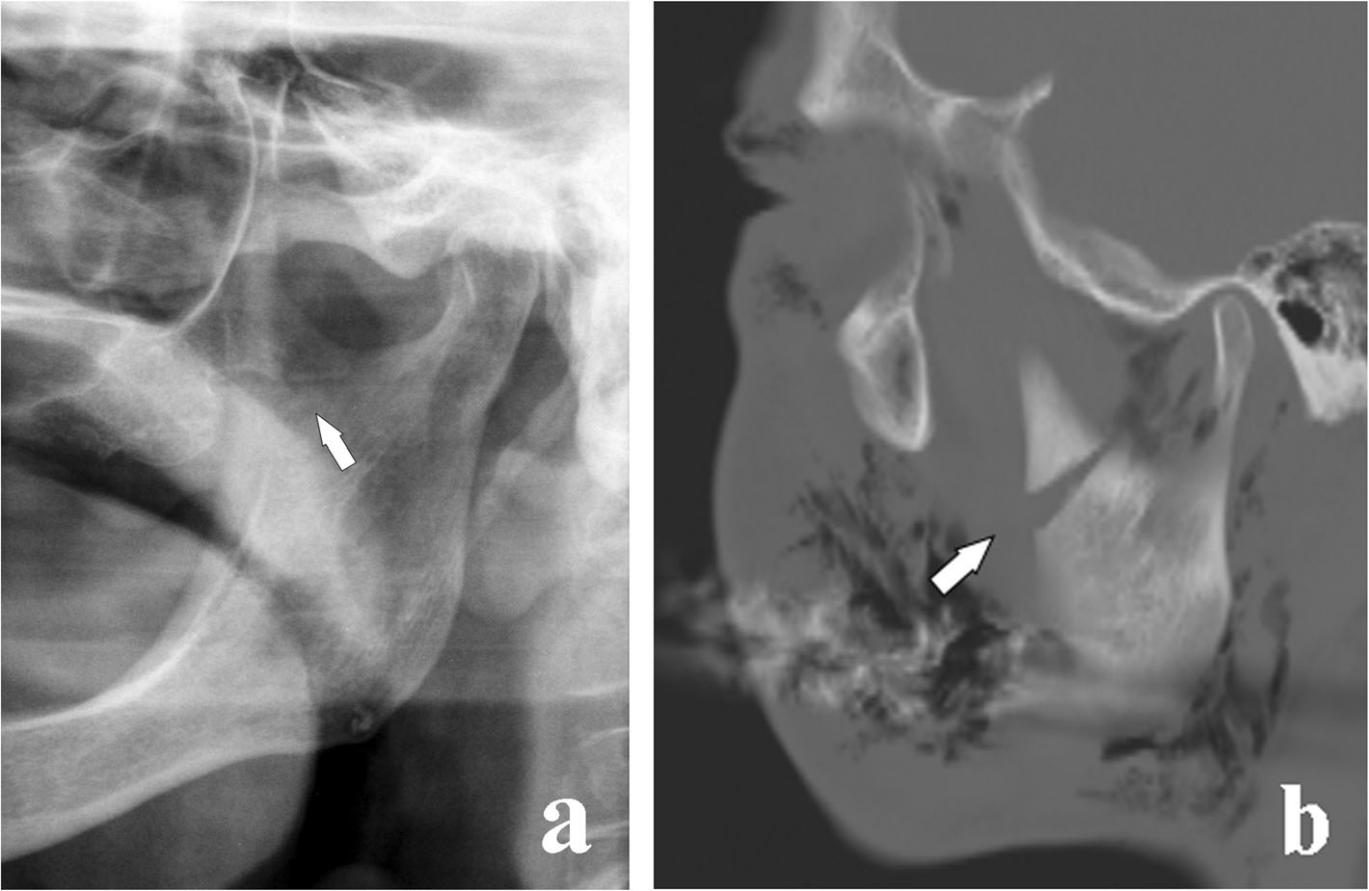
Coronoid process fracture medial to the deepest central point of the sigmoid notch. a Cropped panoramic radiograph in a toothless patient. The fracture rhyme (arrow) originates from the internal face of the coronoid process and ends medially to the deepest central point of the sigmoid notch. b Computed tomography 5-mm oblique reconstruction of a different patient who underwent the examination following a road accident. The displacement of the bone fragment is remarkable (arrow)
